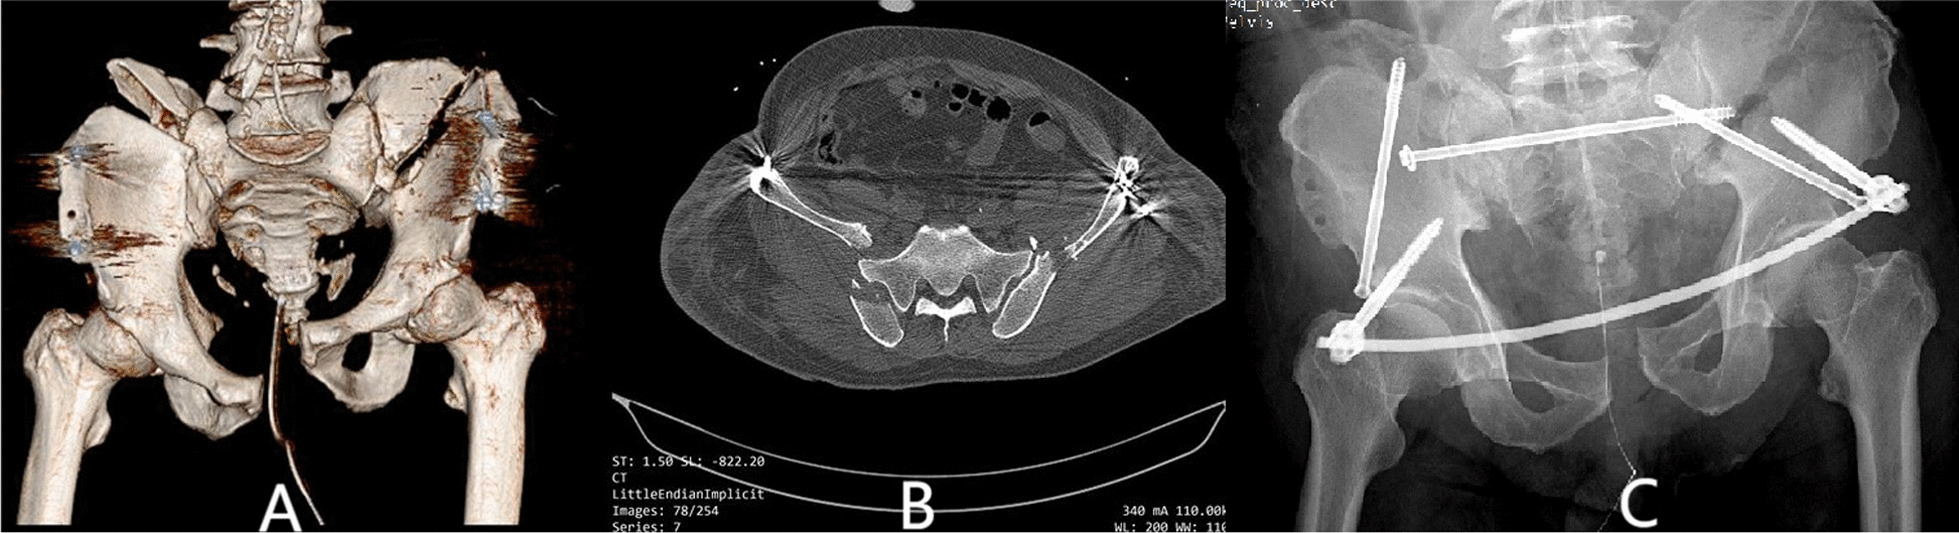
Case 14. A 60-year-old man who was injured from compression underwent S1 transiliac–transsacral screw combined with LC-2 screw fixation for a 301SPD type IIIc fracture. A Preoperative 3d-CT scan shows bilateral sacroiliac joint displacement accompany ilium fracture-displacement. B Preoperative CT scan cross-sectional image shows type II crescent fracture on the right and type I crescent fracture on the left. C The radiological examination image shows that the fracture was reduced and fixed satisfactorily
